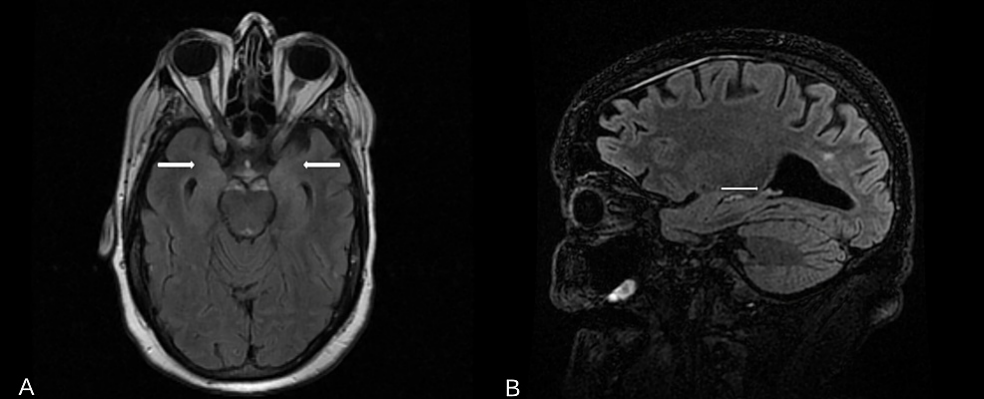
MRI brain with and without contrast T2/FLAIR images. (A) MRI T2/FLAIR axial view and (B) MRI T2/FLAIR sagittal view. FLAIR: fluid attenuated inversion recovery
